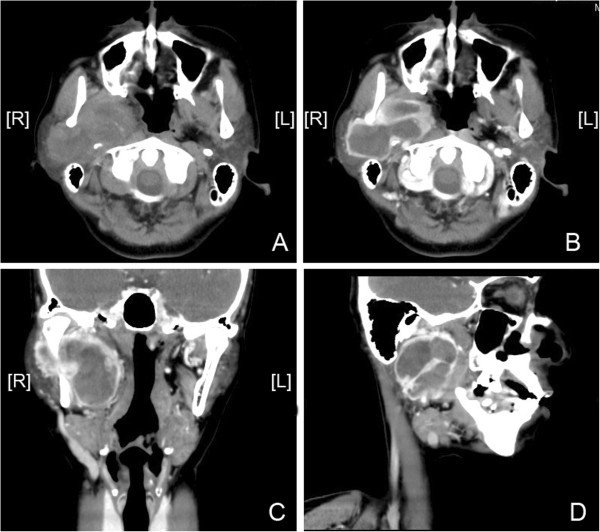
CT images. A is CT plain scan. B, C, D is enhanced CT scan. B is plain scan image, C is coronal scan image and D is sagittal scan image.
